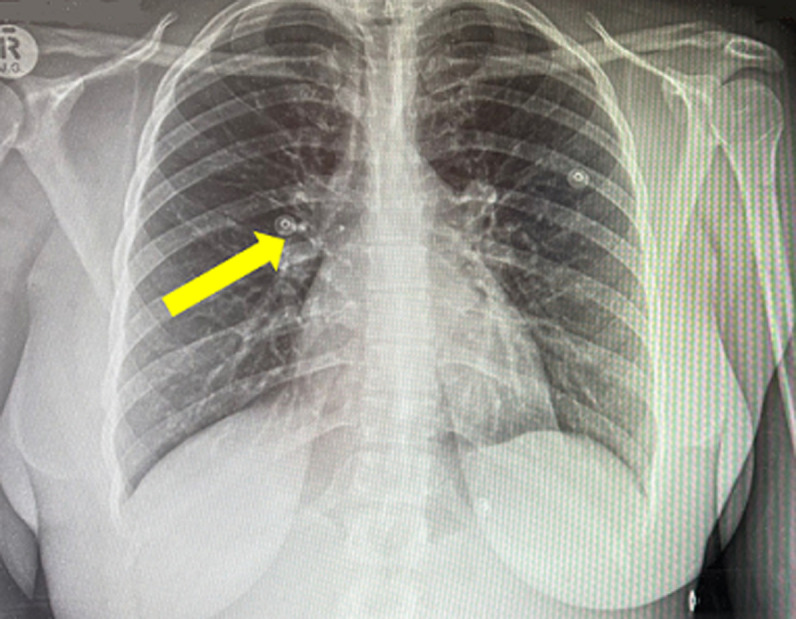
chest X-ray: demonstrating hilar lymphadenopathy (yellow arrow)
